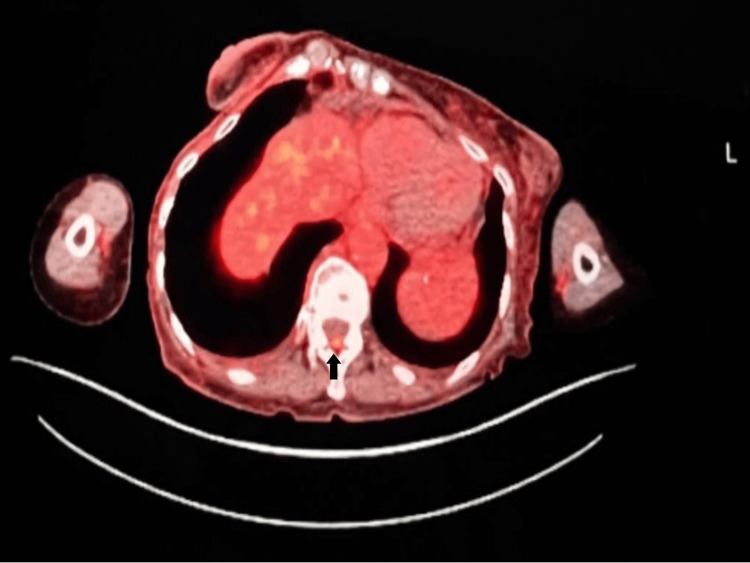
A small focus of abnormal uptake in the T12 posterior epidural space on PET-CT PET-CT: Positron emission tomography–computed tomography.
